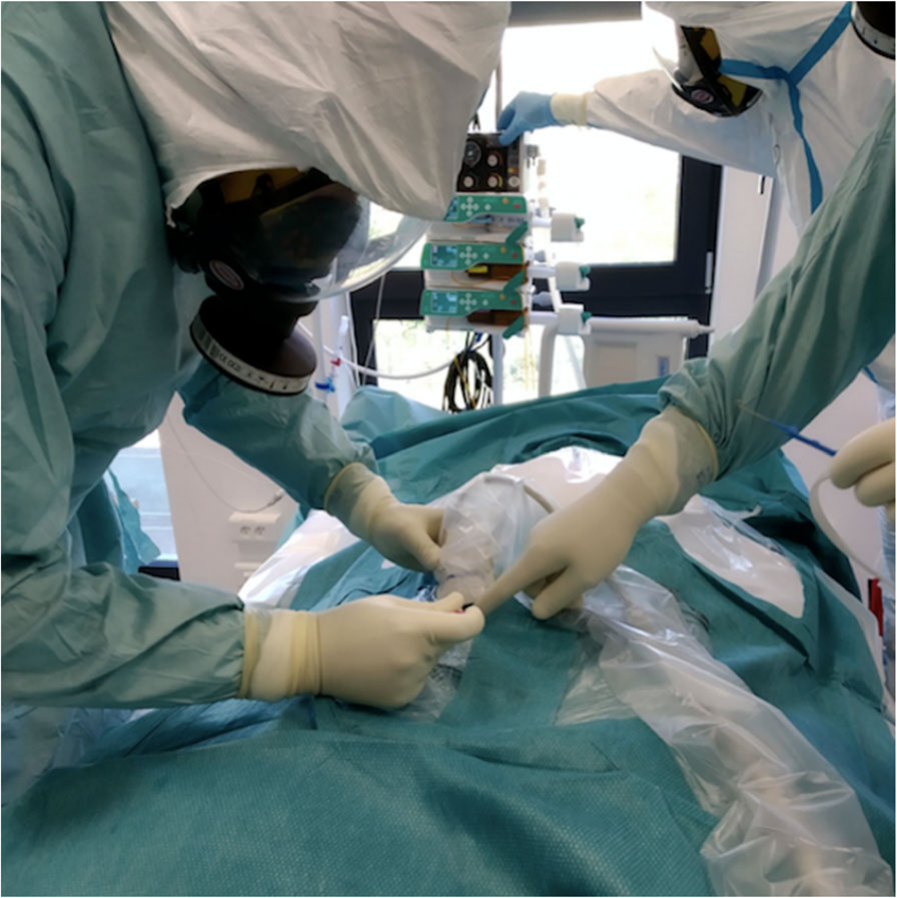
Venous femoral cannulation, percutaneous ultrasound navigated. *(authors’ material)*
